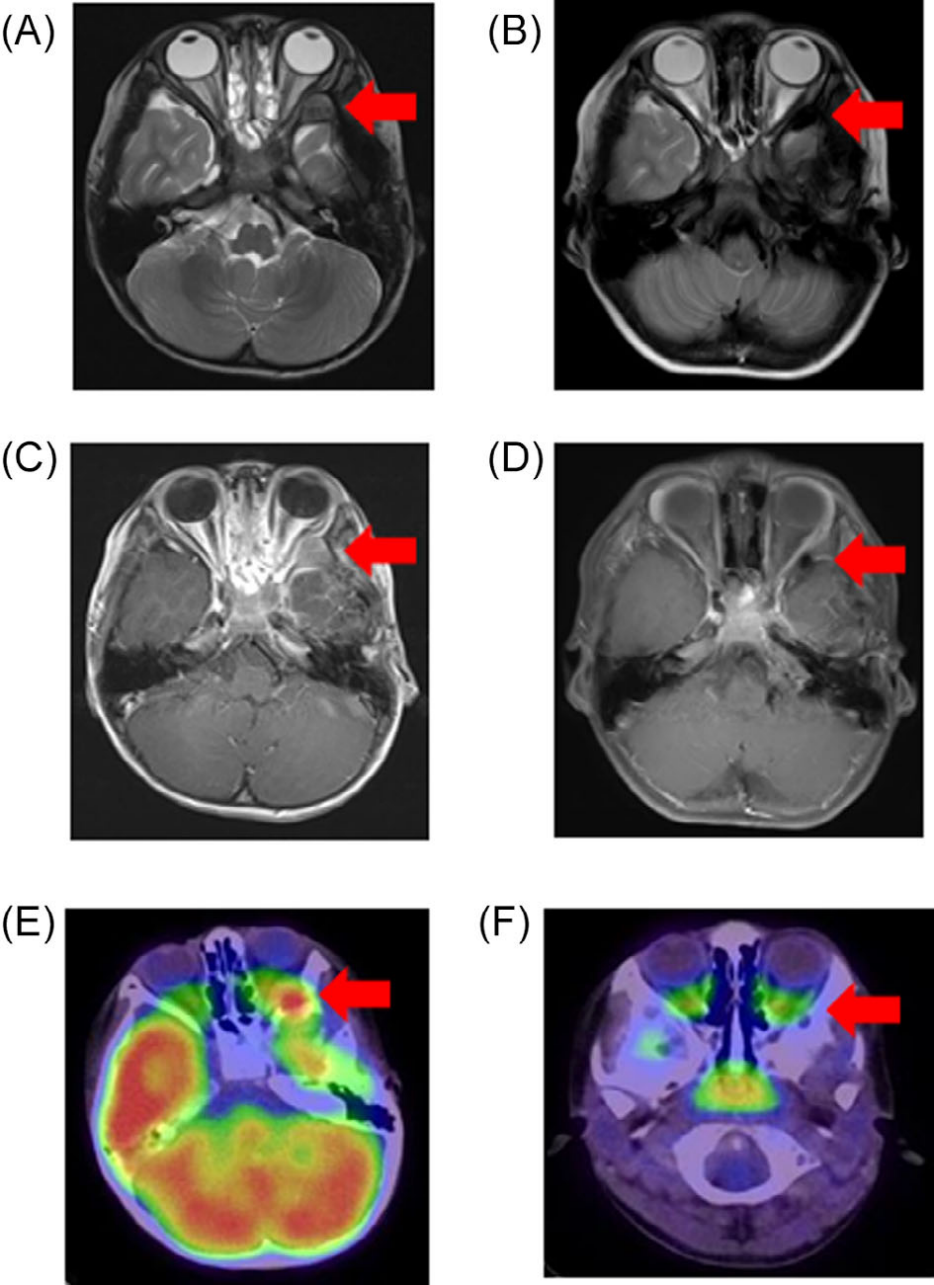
Clinical response to the second administration of CLO at post‐CLO recurrent site in patient 1. Second recurrent site after first completion of CLO is shown by (a) T2‐weighted MRI, (c) T1‐weighted MRI, (e) FDG‐PET before treatment and (b) T2‐weighted MRI, (d) T1‐weighted MRI, (f) FDG‐PET after second administration of six cycles of CLO. Red arrows indicate each site of involved lesions
